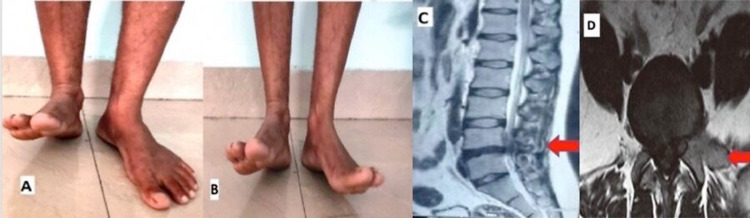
(A) Clinical photograph at the first visit showing left foot drop and (B) at follow up visit showing significant improvement of foot drop. (C) Midsagittal MRI lumbar spine showing L4-L5 PIVD and (D) axial MRI lumbar spine showing protruded disc with foraminal compression PIVD: prolapsed intervertebral disc
